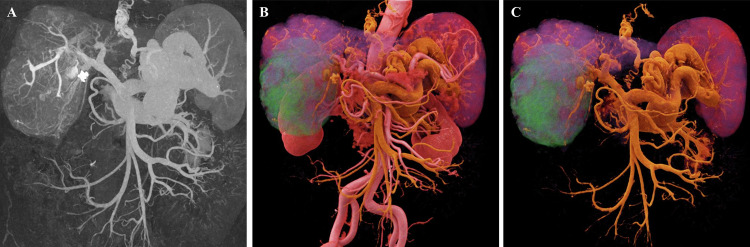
Images of a 62-year-old patient with liver cirrhosis and portal hypertension. A The CTA-based MIP image primarily displays dilated vascular shadows; however, due to vessel overlap, the image lacks spatial depth perception. B, C CR images of the same patient provide a stereoscopic view of the tortuous and dilated morphology of the portal vein, gastric fundal veins, and splenic vein. By masking the obscuring aortic structure, the anatomical details of the splenorenal shunt are intuitively revealed, providing a precise three-dimensional reference for the clinical evaluation of collateral circulation
